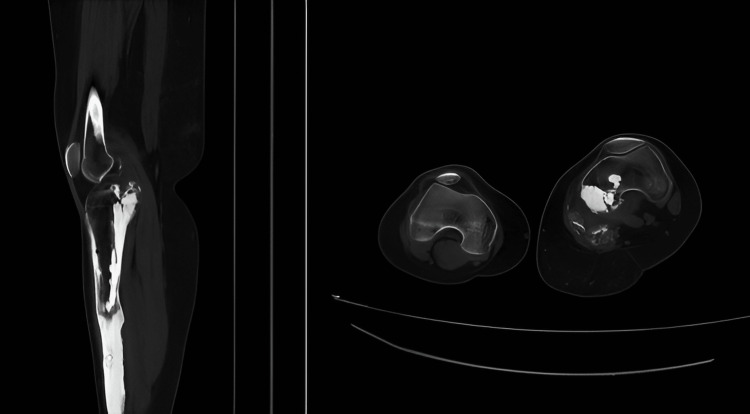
The flow of melted wax appearance - melorheostosis - around the left knee on axial and sagittal sections of the CT scan.
